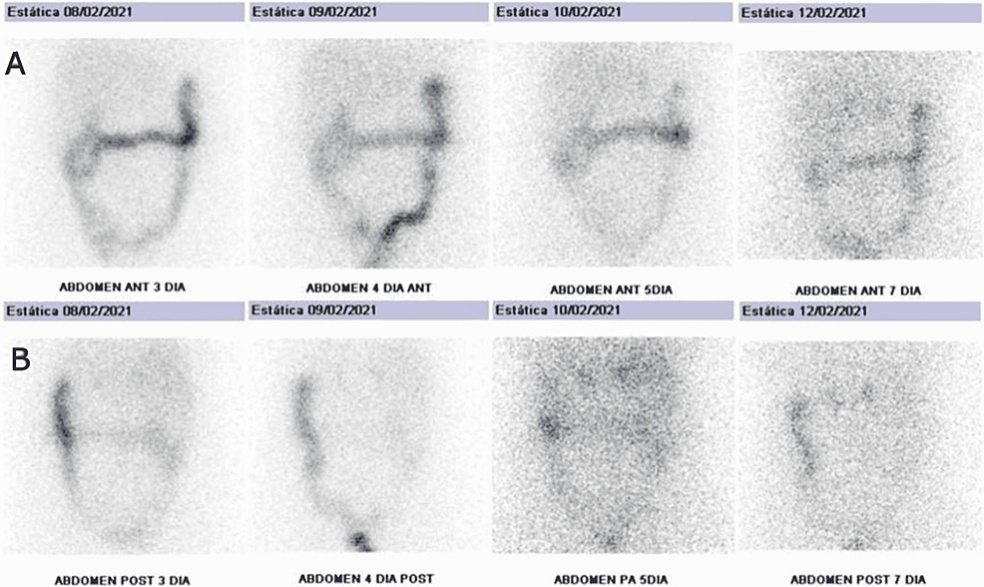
A: First phase of the scintigraphy with uptake predominantly in the colon. B: Second phase of the scintigraphy with uptake predominantly in the right adrenal gland.
